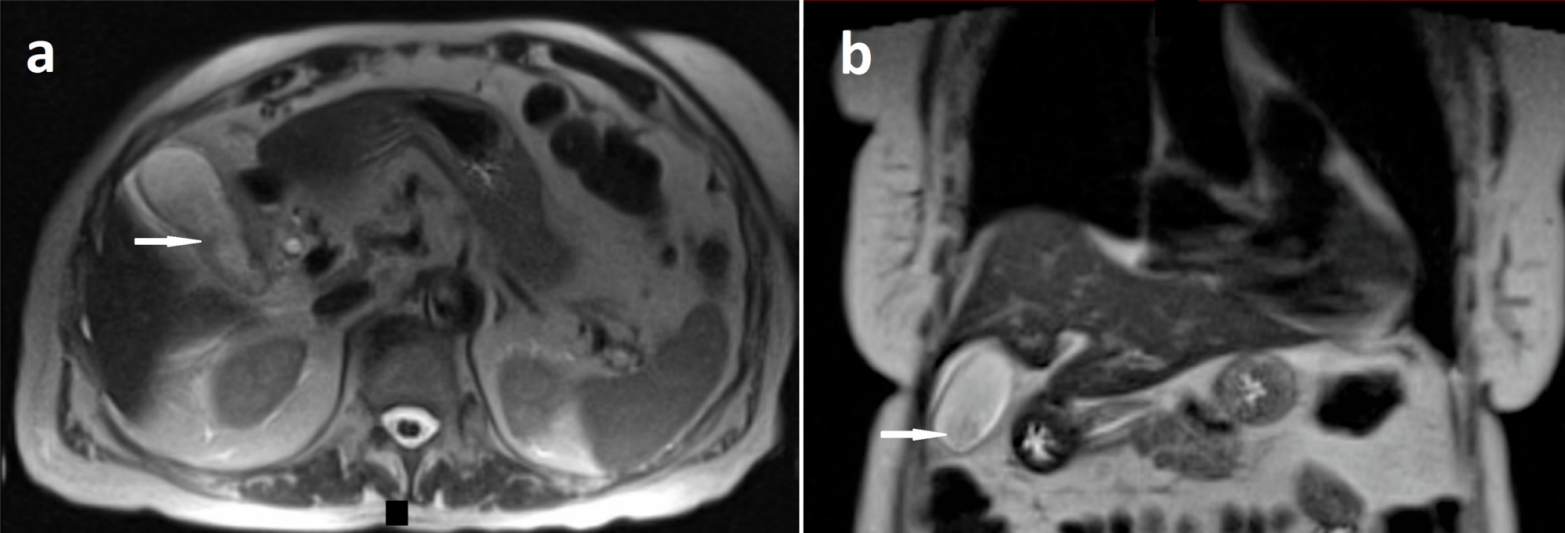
Magnetic resonance imaging (MRI) of the abdomen showing sludge in the gallbladder Axial (a) and coronal (b) sections of T2-weighted contrast-enhanced abdominal MRI showing layering sludge in the gallbladder (white arrows) with gall bladder wall thickening and trace pericholecystic fluid.
